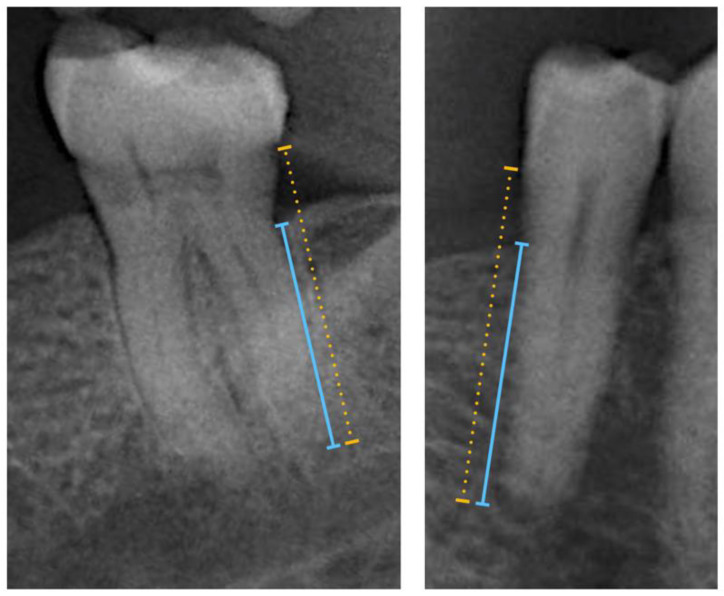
Radiographic-based Periodontal Bone Loss (R-PBL) was measured from the marginal alveolar bone to the tooth apex (blue line) and from the cementoenamel junction to the tooth apex (yellow dotted line), as in Rydén et al. [16] The examples show R-PBL measurement in a molar and premolar.
